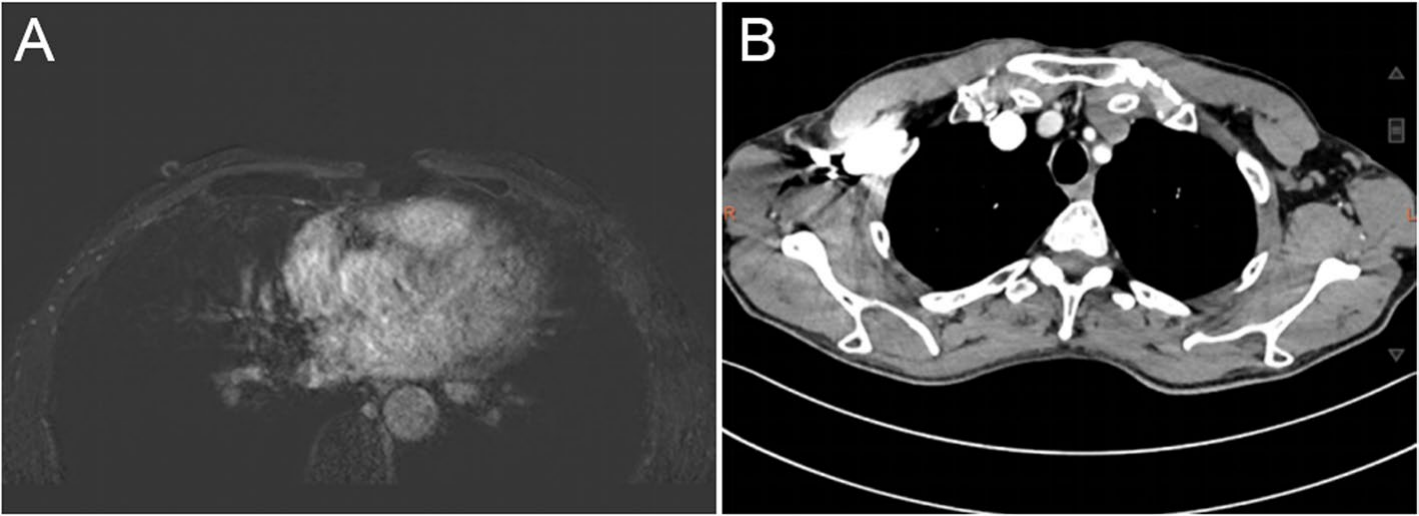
Imaging examination findings of a patient with sweat gland cancer. A Breast MRI showed no abnormal mass. B CT showed no enlarged lymph nodes in the left armpit
